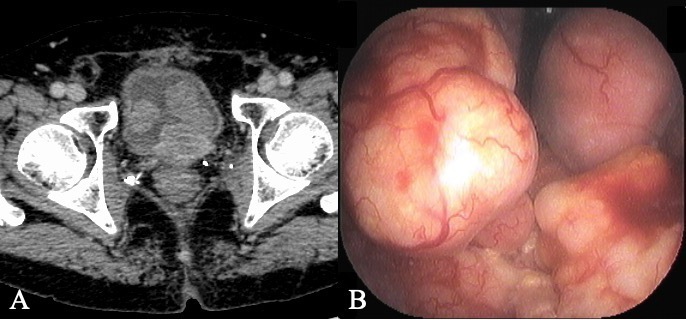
(A) CT shows tumor recurrence in the prostate bed with multiple
